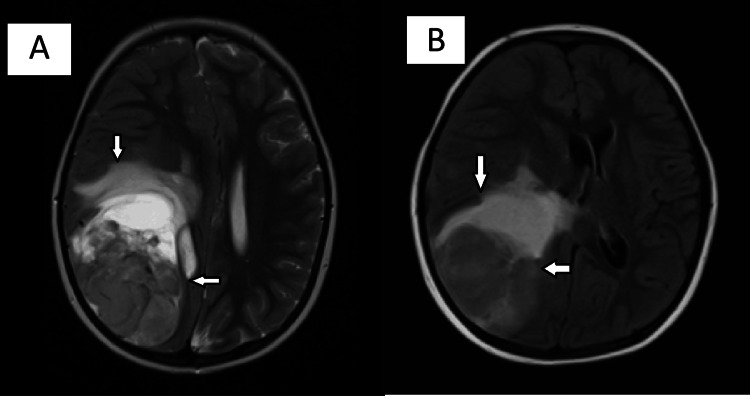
Contrast-enhanced MRI of the brain. (A) T2 contrast axial sections showing heterogeneously hyperintense intra-axial mass lesion measuring 70 x 49 x 60 mm, featuring a cystic area on its anteromedial aspect associated with perilesional edema. (B) T2 FLAIR axial sections showing heterogeneously hyperintense intra-axial lesion with cystic area MRI: magnetic resonance imaging; FLAIR: fluid-attenuated inversion recovery
